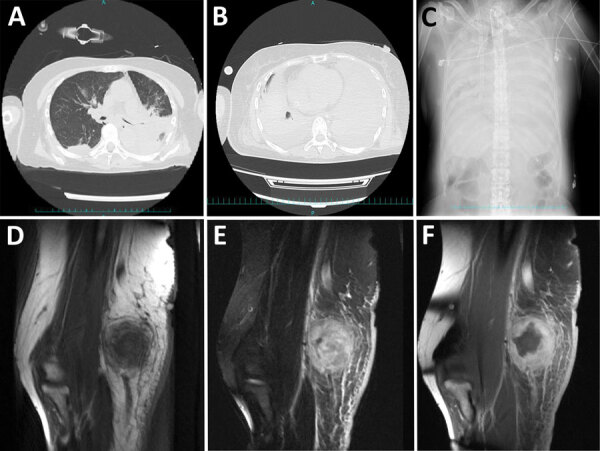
Computed tomography, radiograph, and magnetic resonance imaging results for patients with *Corynebacterium ulcerans* infection, Japan, 2001–2020. A–C) Chest computed tomography images and radiograph of patients with severe respiratory symptoms. Atelectasis noted at admission (A, top) and after exacerbation of symptoms (B, bottom) (case no. 29, from Dr. Hayashi, Maebashi Red Cross Hospital, Gunma, Japan). Spread of atelectasis noted on chest radiograph taken at the time of exacerbation of symptoms (C) (case no. 29, also from Dr. Hayashi, Maebashi Red Cross Hospital). D–F) Magnetic resonance imaging of an elbow abscess. Magnetic resonance imaging T1-weighted images show equal brightness to the muscles (D), fat-suppressed T2-weighted image by short-tau inversion recovery method show unevenly high brightness (E), and contrast-enhanced T1-weighted images show a mass whose margins are contrast-enhanced (F) (case no. 10, from Dr. Urakawa, Tsuruoka Municipal Shonai Hospital, Yamagata, Japan).
